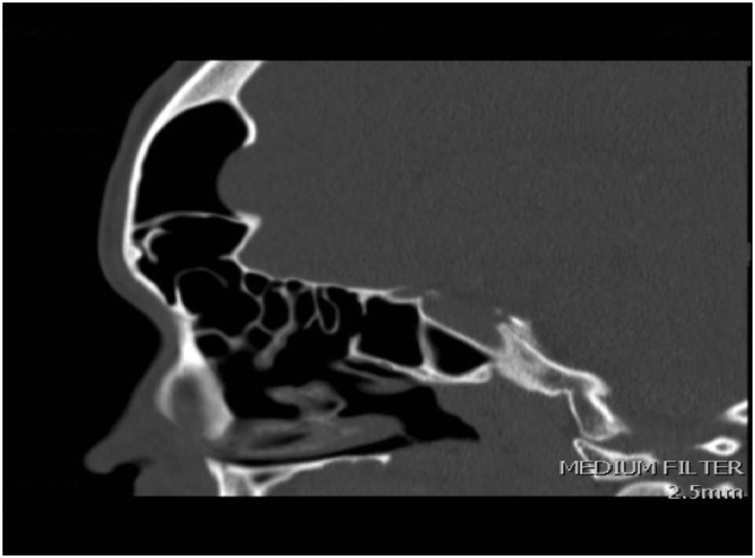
Post op Sagittal CT sinus.
